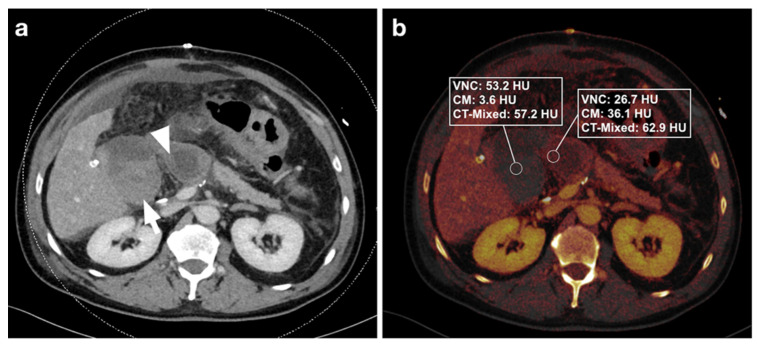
(a) 59-year-old male patient with intra-abdominal hematoma (arrow) adjacent to small bowel loops (arrowhead) after Whipple surgery for pancreatic cancer. (b) Measurements in iodine maps show a difference in VNC and iodine values (dual-energy CT) between hematoma and intestinal loops, but no difference in DE-LB (single-energy CT). After CT, the patient was reoperated, and the hematoma was removed.
